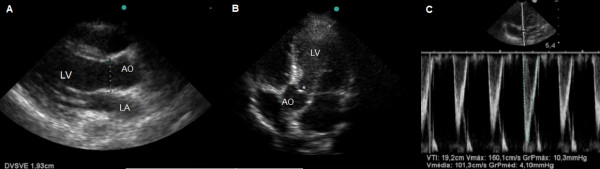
Cardiac index calculation. A- Measurement of the left ventricle outflow tract by the parasternal long-axis view (diameter of the aortic annulus during systole). B and C- Measurements of aortic VTI through pulsed wave sample volume (*) at the aortic annulus (apical 5-chamber view). Ao, aorta; LA, left atrium; LV, left ventricle.
